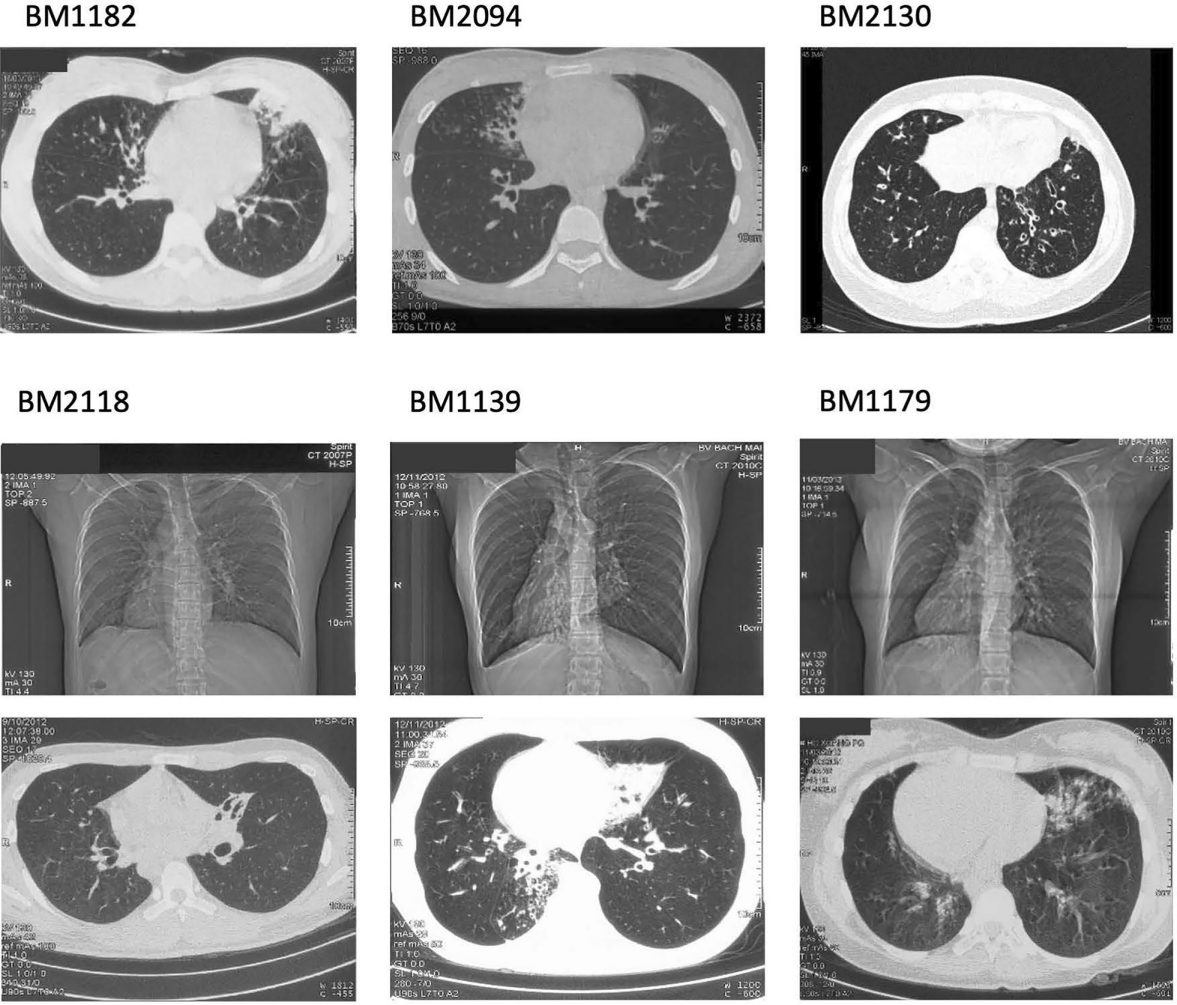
Chest CT images of patients with genetically confirmed CF or PCD, and with situs inversus. Patient BM1182 carried pathogenic *CFTR* variants. Patients BM2094, BM2130, and BM2118 had PCD-causing gene variants. Patients BM2118, BM1139, and BM1179 exhibited situs inversus. *CT* computed tomography, *CF* cystic fibrosis, *PCD* primary ciliary dyskinesia
